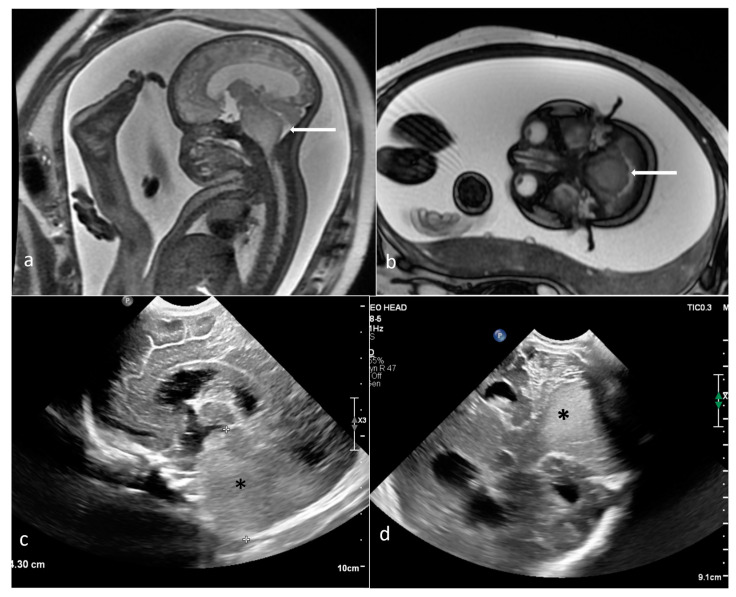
Diffuse brain stem glioma. Fetal MRI (arrows): (a) sagittal T2WI; (b) axial FIESTA. (c,d) US images of a brain stem mass reaching through the mastoid fontanelle after birth (asteriks).
